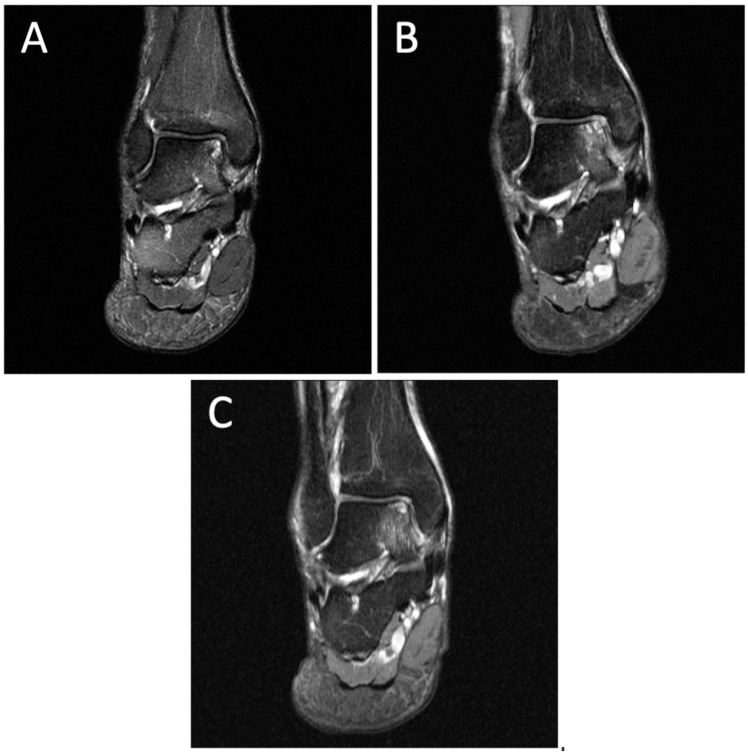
Degenerative progression of the right medial osteochondral lesion on coronal MRIs from 2011 to 2021. The timeline of OLT progression includes MRI scans from 2011 (A), 2015 (B), and 2021 (C).
